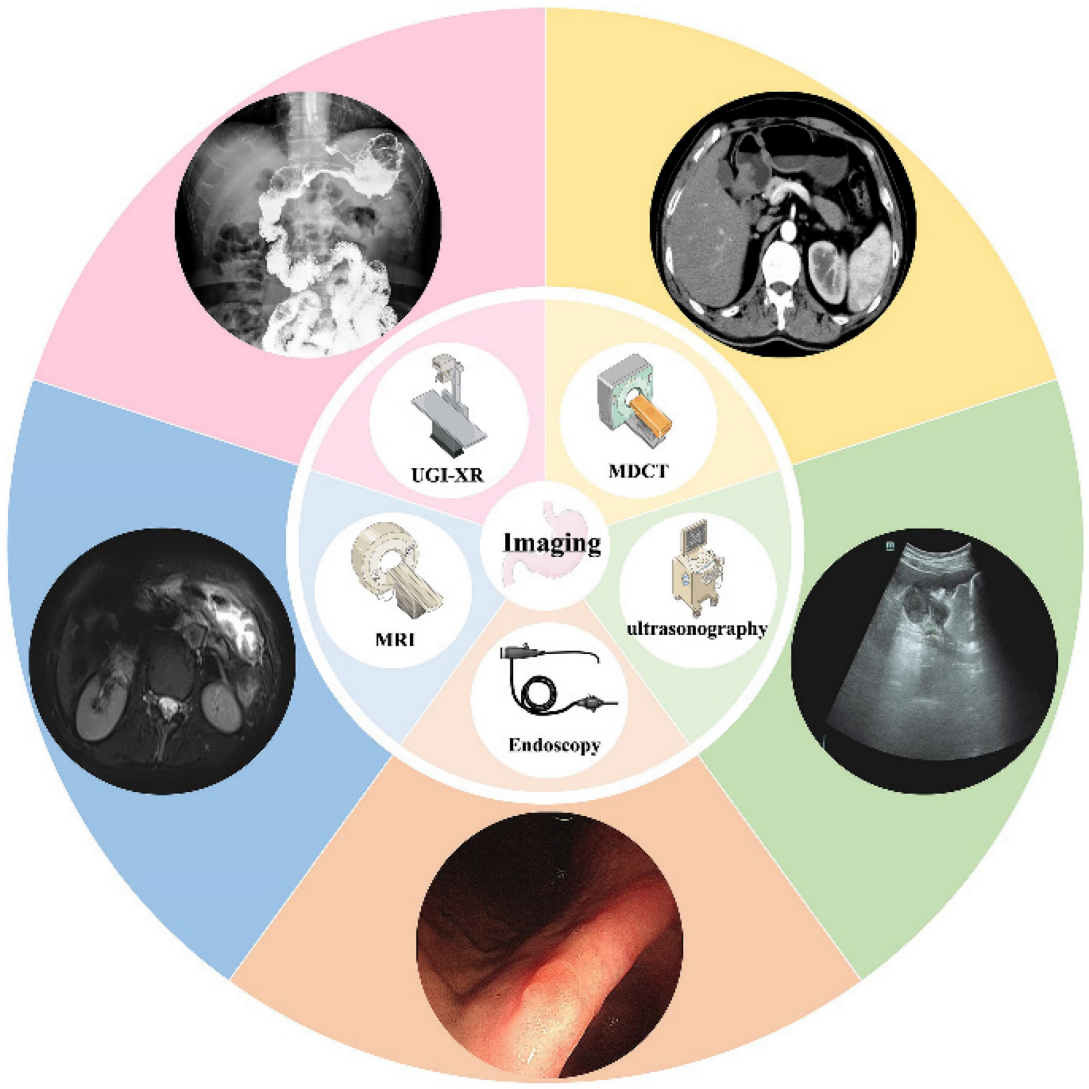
Imaging techniques of gastric cancer screening. Including UGI, MRI, gastric ultrasonography, and endoscopy.
